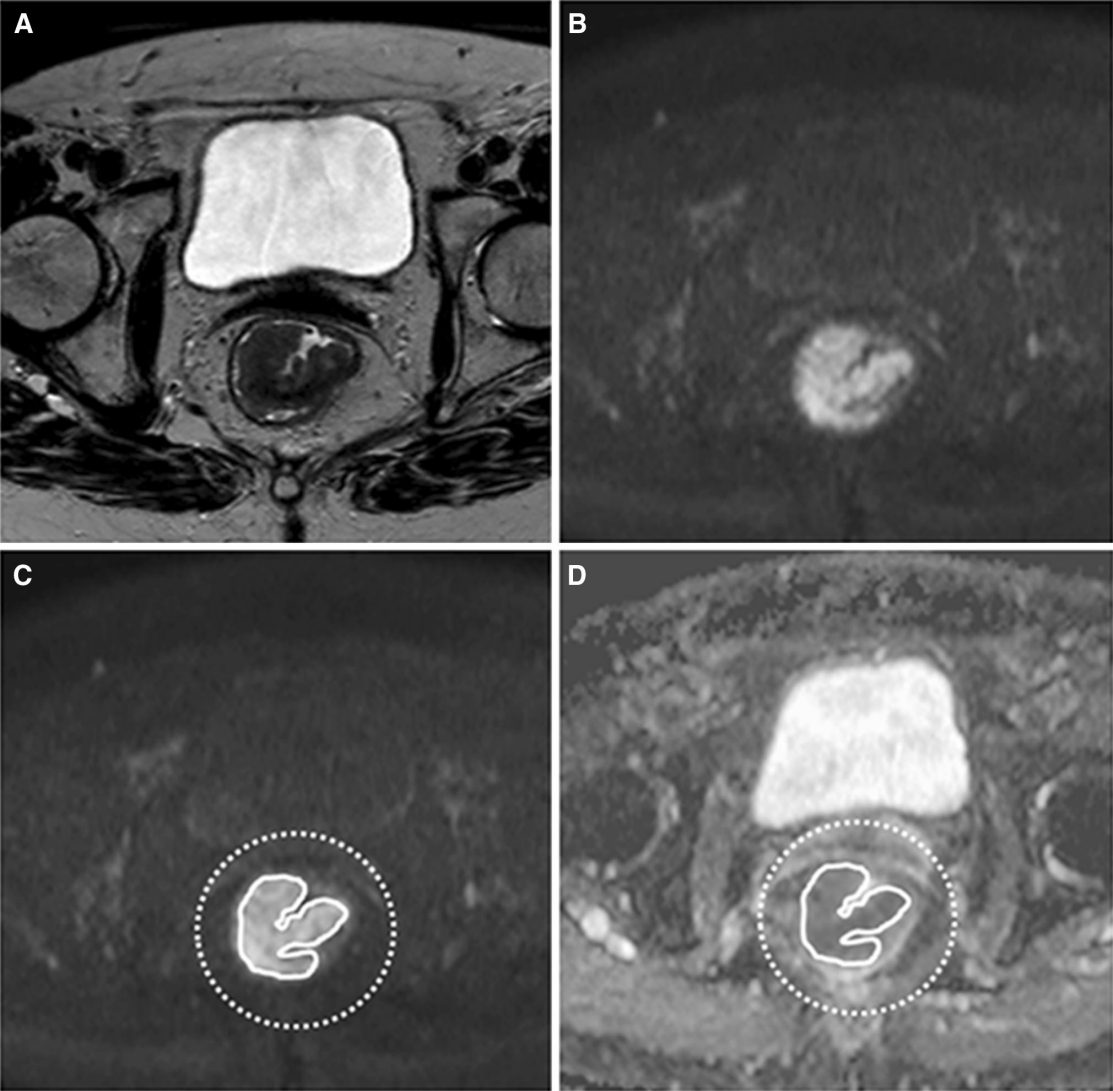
Example of the MR exam of an 82-year-old female patient. A Axial T2W image shows a semi-circular tumor, B the b-1000 DWI shows high signal in the tumor area, C example of the precise delineation (*solid line* performed by reader 1) and the non-precise delineation (*dotted line* performed by reader 3), the latter including both tumor and surrounding tissues, and D both delineations transferred to the ADC map
